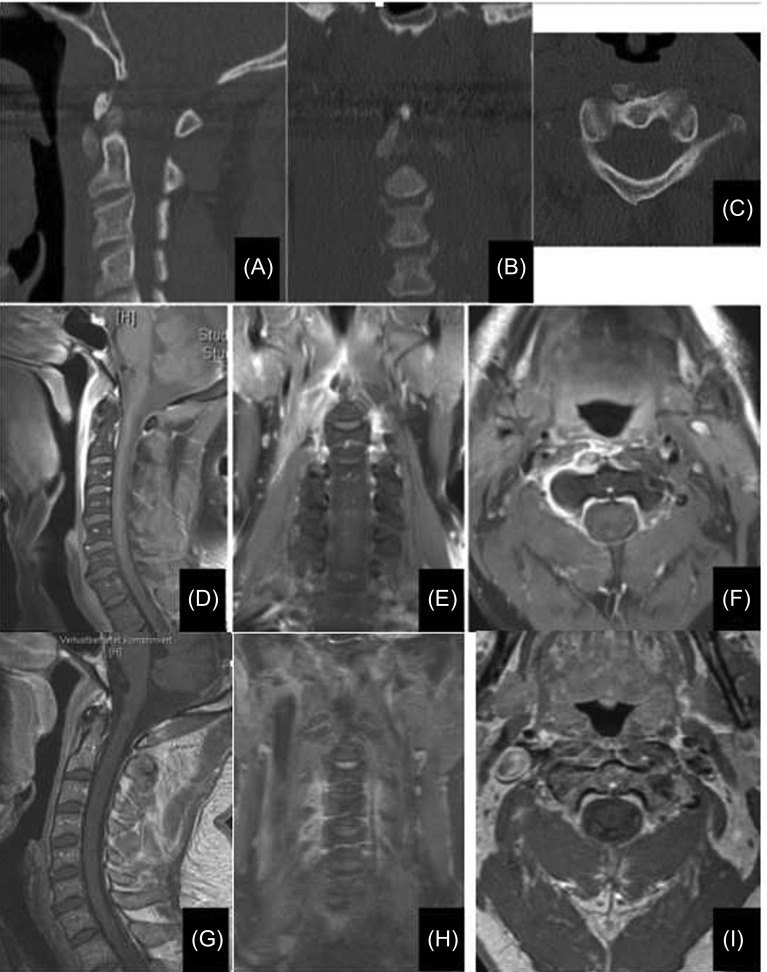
(A–C) CT scans showing calcifications at longus colli origin anterior to C1/2, measuring about 14 mm in the superior-inferior diameter and about 7 mm in the anteroposterior diameter. (D–F) Prevertebral swelling and minimal fluid collection extending from skull base caudally to C4, maximally at the middle of C2 (up to 7 mm). Musculus longus colli on the right side is edematous especially at its insertion at C1 and C2 vertebrae. No other sites of inflammation in the ENT area. The findings correspond to right-sided longus colli tendinitis. (G–I) A follow-up MRI with the same sequences shows the obvious regression of the soft tissue swelling as well as the contrast enhancement. *Note*: the MRIs were done using two different devices and the mostly corresponding cuts were selected for the comparison between that at presentation and at follow-up.
